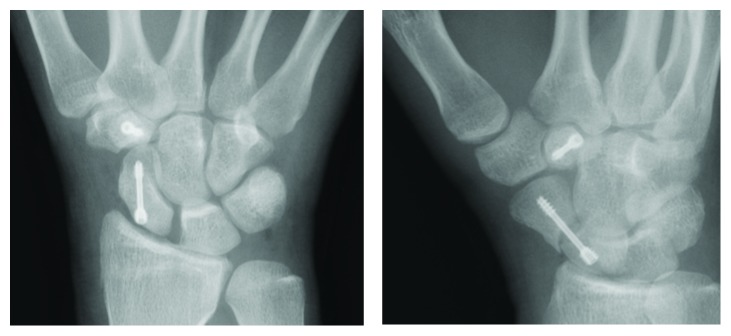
Postoperative X-ray films showing internal fixation with headless bone screws.
